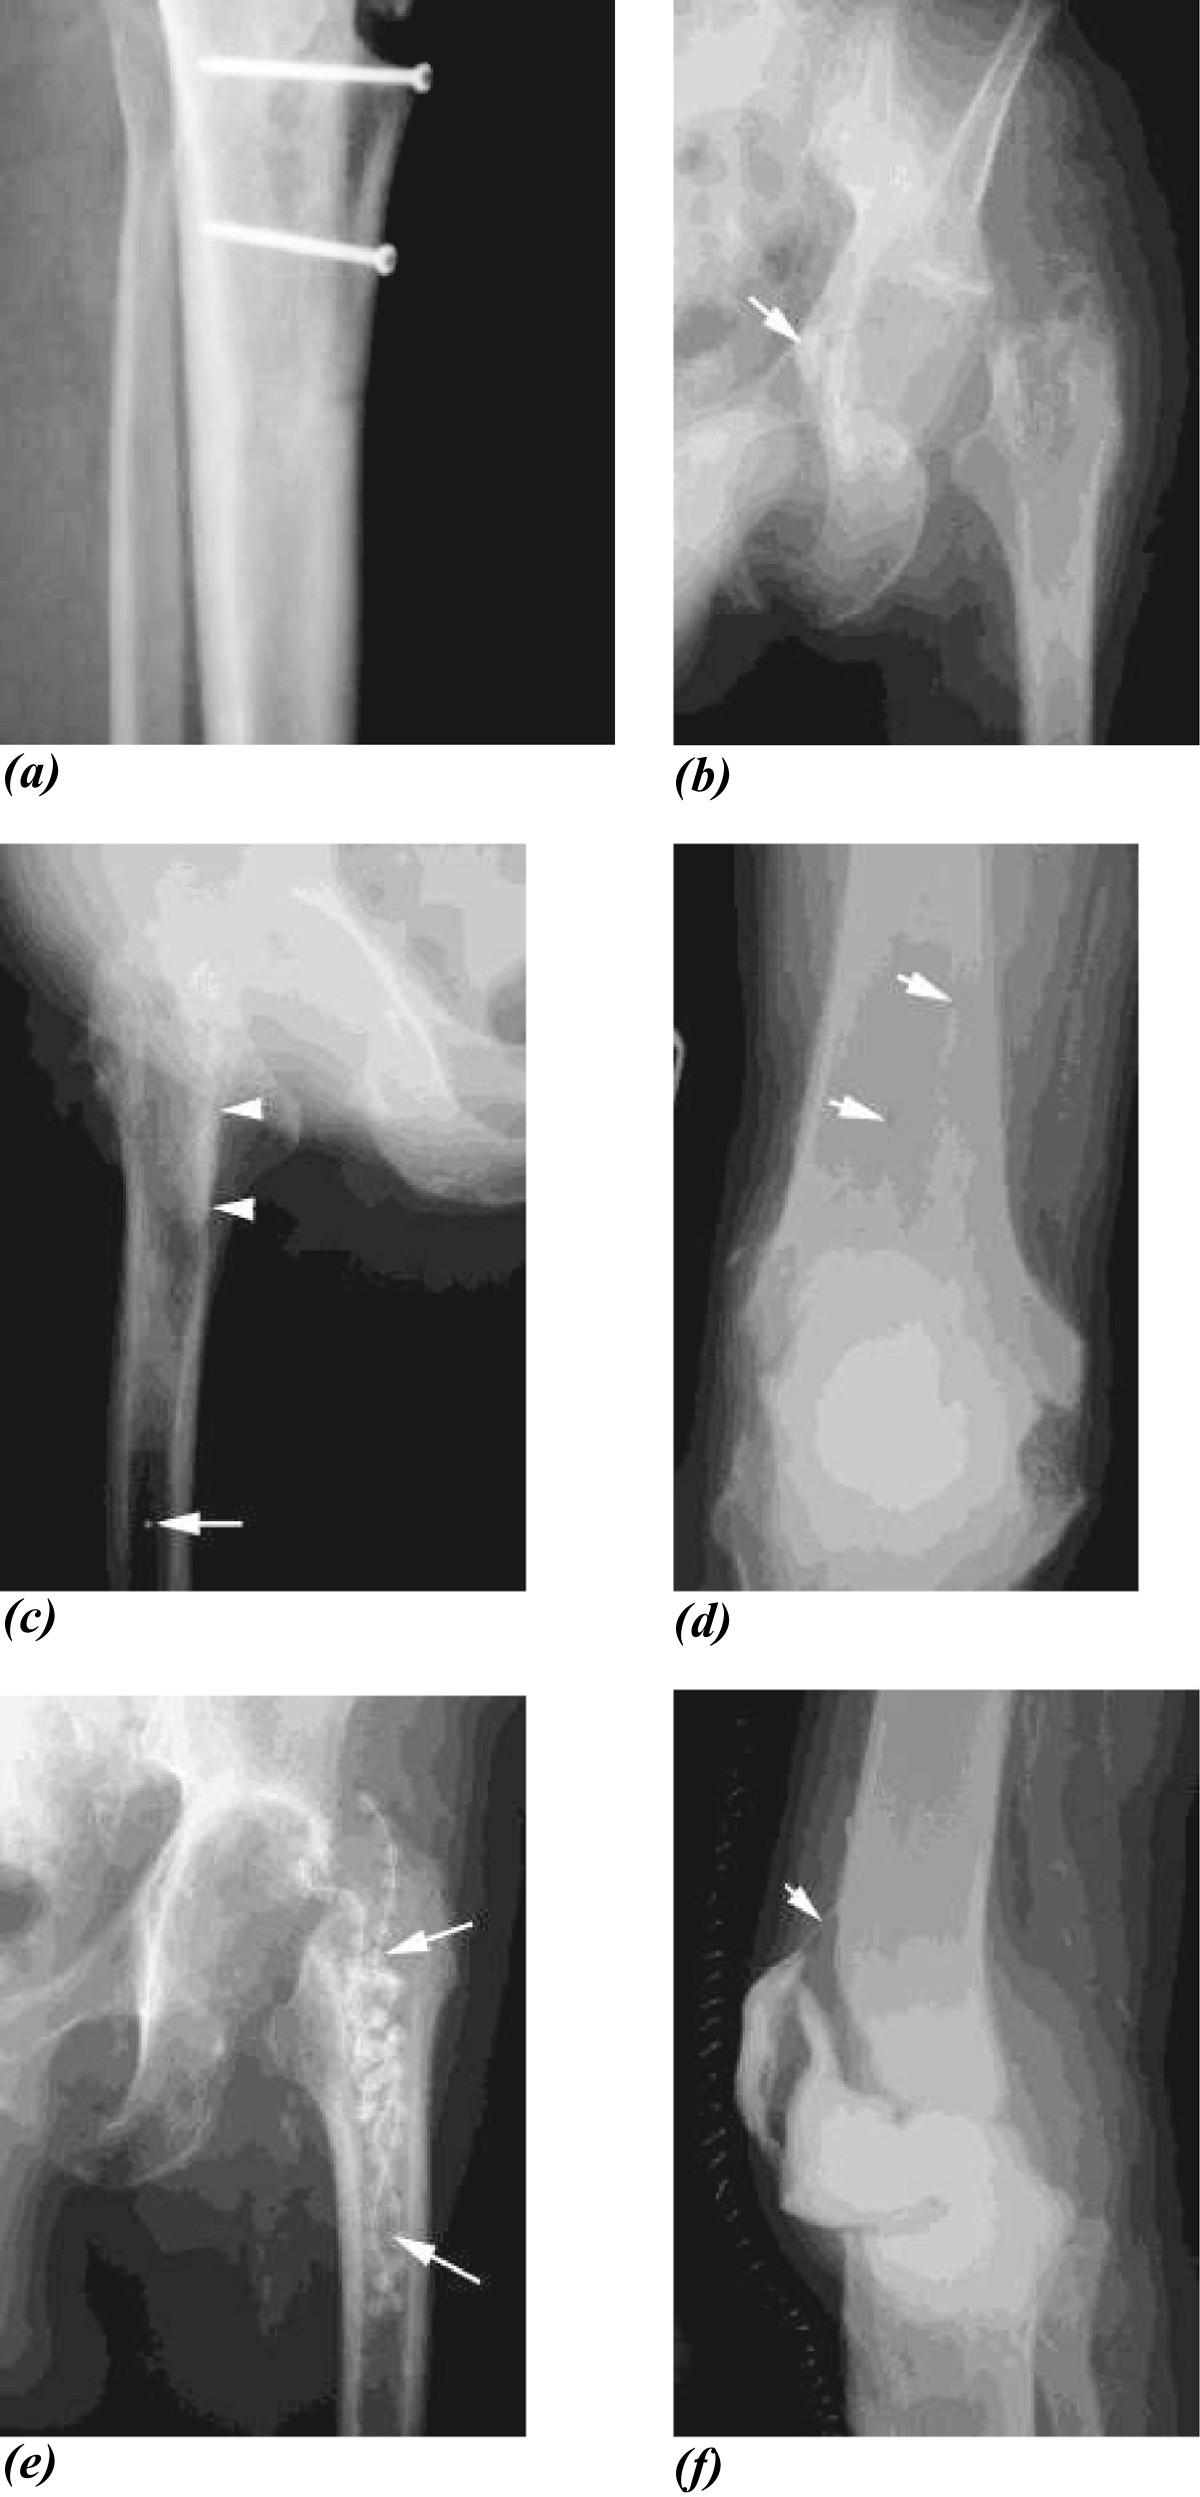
Post first-stage radiographs were examined for the presence or absence of the following seven features: retained metalwork (not shown), new metalwork (Figure
